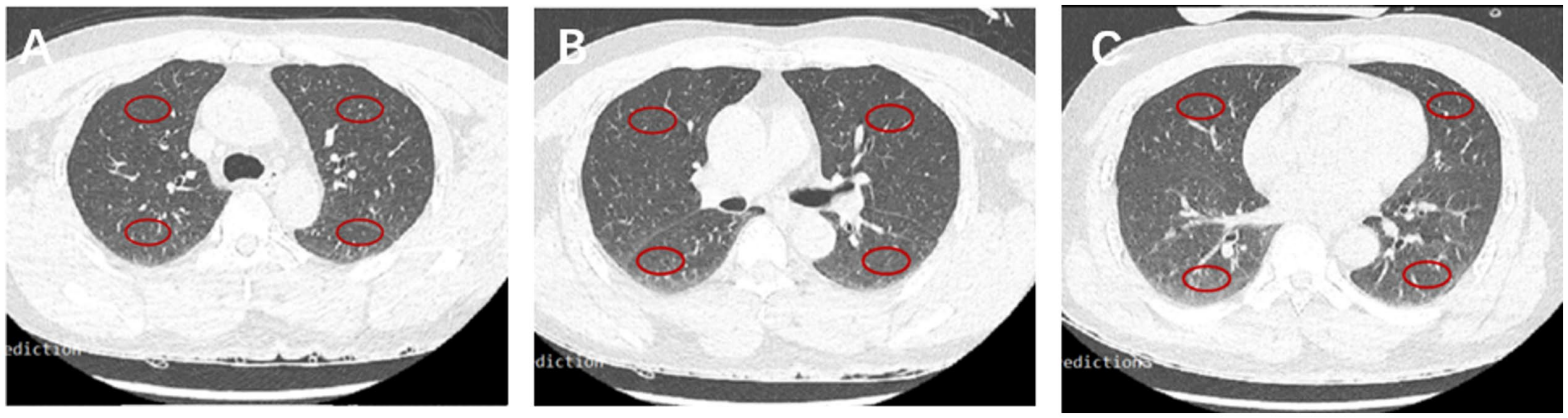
CT HU in 12 fields of the lung. Representative ROIs in different lobes in the carina (A), main stem bronchi (B), and bottom (C) levels.
